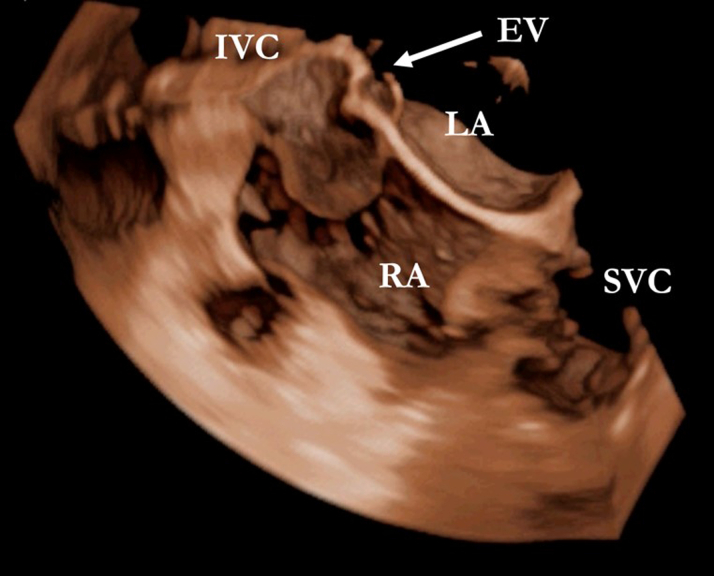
Transesophageal Echocardiogram With a 3-Dimensional Image Showing the Eustachian Valve Entrapped in the Atrial Septum
